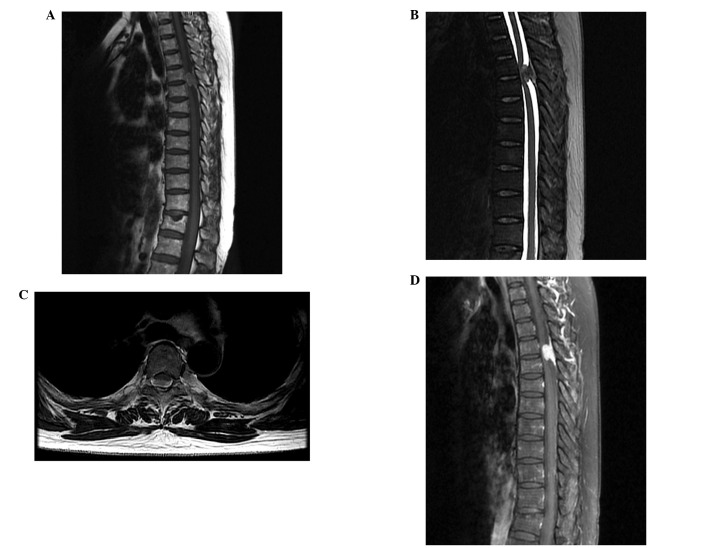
(A–C) Preoperative magnetic resonance imaging showing characteristics of a T4–T5 intradural extramedullary spinal mass. (A) T1-weighted image showing the hyperintense signal lesion. (B and C) T2-weighted images showing a hyperintense signal on (B) sagittal and (C) transaxial images. (D) T1-weighted image with contrast medium showing homogeneous enhancement of the lesion with the dural tail sign.
